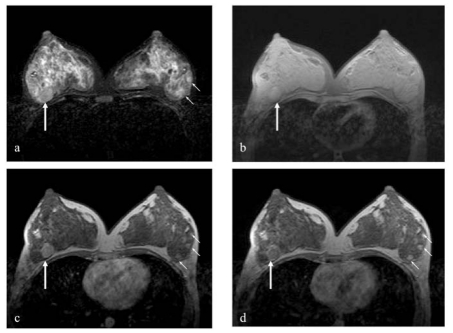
MRI study of the breasts showing the medullary carcinoma in the right breast adjacent to the pectoralis muscle (long white arrow) appearing (a) isointense on T2W STIR axial (TR: 5450, TE: 60), (b) isointense on T1W fat sat axial sequence (TR: 11.2, TE: 5), (c) demonstrated homogenous enhancement during the early dynamic post-gadolinium sequence at 1 minute, and (d) delayed peripheral enhancement during the dynamic post-gadolinium at 6 minutes, suggestive of a malignant lesion. Note the smaller three enhancing fibroadenomas in the left breast (short white arrows) in (c) and (d), which demonstrated gradual homogenous enhancement throughout the dynamic phases.
